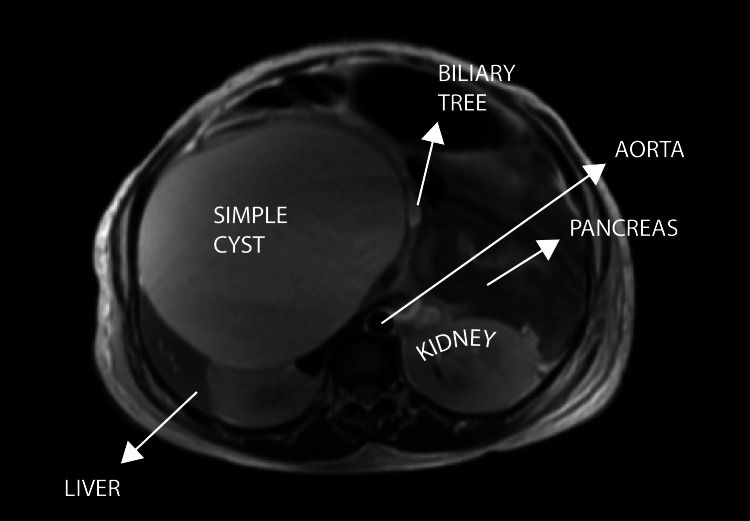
Axial T2-weighted MRI The image shows a cyst compressing the right kidney (inferiorly) and indenting and displacing the head of the pancreas (medially) MRI: magnetic resonance imaging
